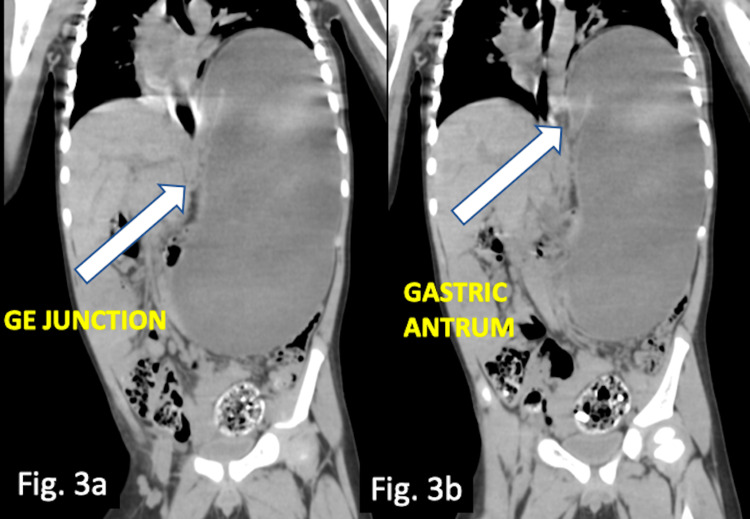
Non-contrast CT abdomen coronal sections showing (a) overdistended and (b) upside-down stomach.
